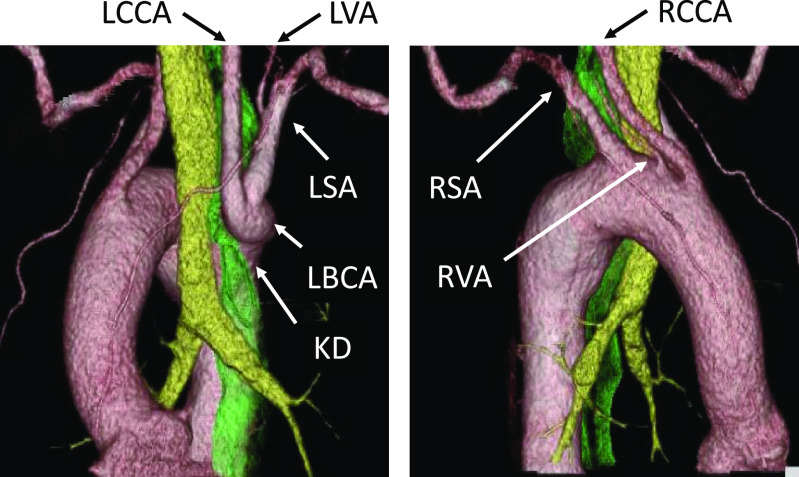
Three-dimensional computed tomography showing the right-side aortic arch branches from proximal to distal in the following order: right common carotid artery (RCCA), aberrant right vertebral artery (RVA), right subclavian artery (RSA), and the aneurysmal Kommerell diverticulum (KD). The KD connects to the left common carotid artery (LCCA), branching into the common carotid artery (LCCA) and the left subclavian artery (LSA). The esophagus (dark green) is compressed by the trachea (light green), and the KD connects to the LCCA.
